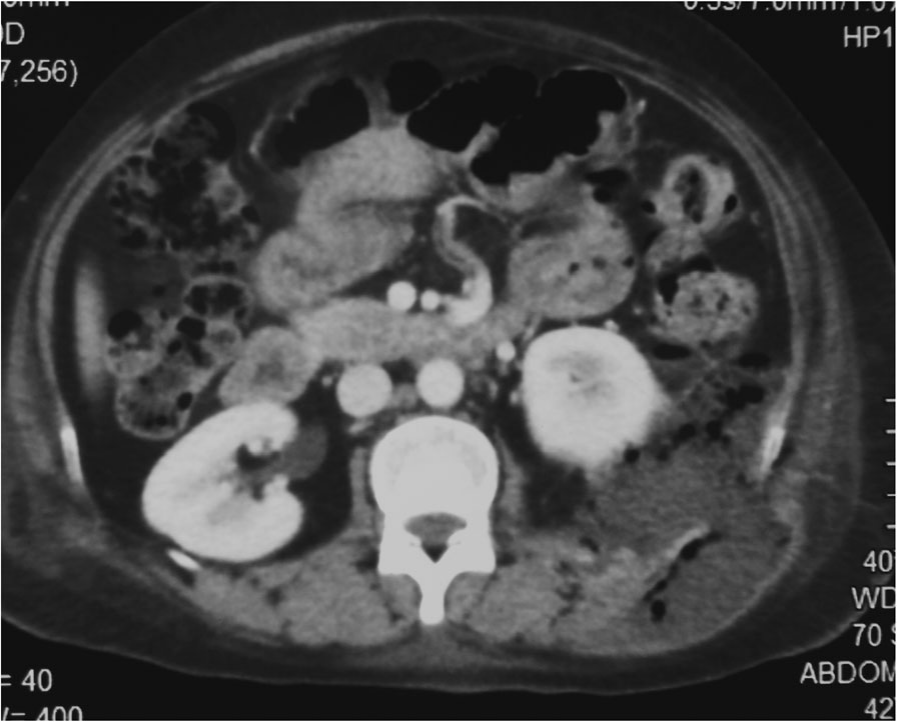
Contrast enhance CT abdomen showing a retroperitoneal abscess behind the left kidney which had extended to subcutaneous tissue plane through a defect in the posterior abdominal wall
